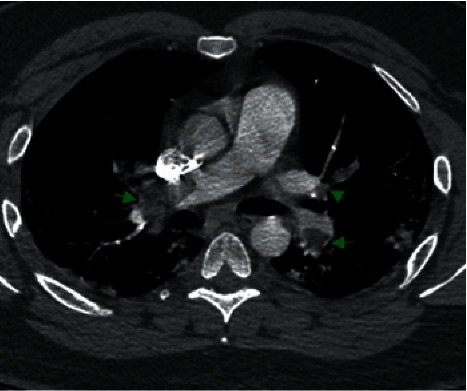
Chest axial CT image on a vascular window shows extensive bilateral multifocal acute pulmonary embolism (green arrows) without any radiological evidence of right heart strain.
